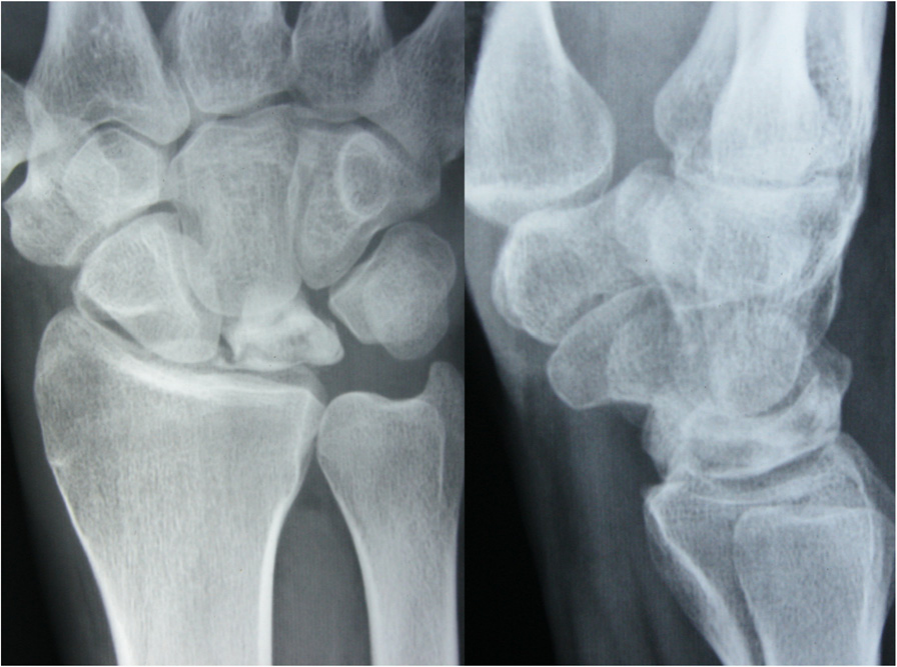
Preoperative wrist radiographs of stage II Kienböck’s disease.
